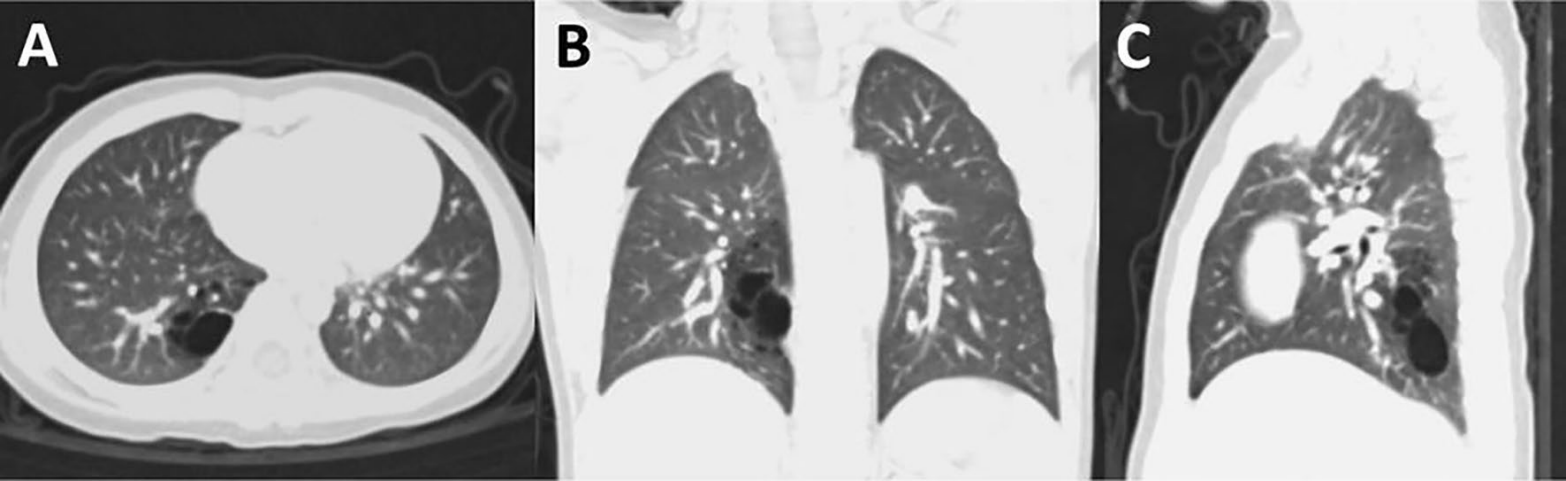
Congenital pulmonary airway malformation limited to the posterior basal segment (S10) of the right lower lobe on a chest computed tomography scan. (A) axial view (B) coronal view (C) sagittal view.
